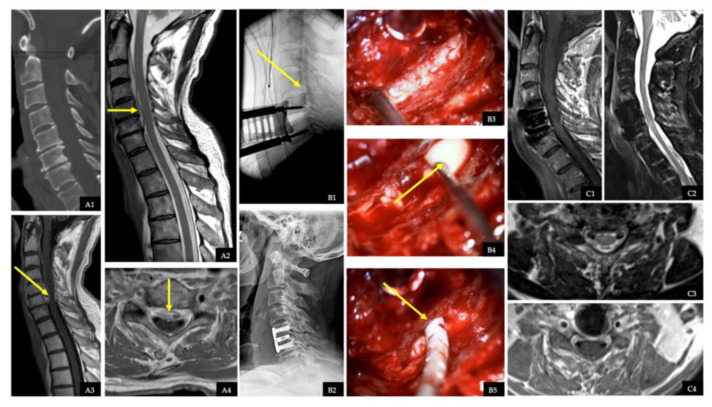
Clinical management of spinal epidural abscess. (A1): Preoperative sagittal reformatted CT image showing no sign of spondylodiscitis. (A2): Preoperative sagittal T2-weighted MR image of the cervical spine showing an isolated spinal epidural abscess (SEA) on the ventral part of the spinal canal at the level of C2 to C7 (yellow arrow). (A3,A4): Preoperative sagittal and axial contrast-enhanced T1-weighted MR images presenting the SEA (yellow arrows). (C3,C4): Preoperative sagittal and axial contrast-enhanced T1-weighted MR images of the cervical spine showing an SEA (yellow arrows). (B1): Intraoperative sagittal X-ray showing the insertion of epidural drainage from the C5/C6 level to C2 (yellow arrow). (B2): Postoperative sagittal X-ray image showing the anterior cervical discectomy and fusion (ACDF). (B3): Intraoperative image presenting the situation after discectomy of C5/C6 without signs of infection. (B4): Intraoperative image showing the pus outflow from the posterior longitudinal ligament after opening it (yellow arrow). (B5): Intraoperative image revealing the insertion of the catheter up to the level of C2 (yellow arrow). (C1–C4) Sagittal and axial T2-weighted and contrast-enhanced T1-weighted MR images at the time of discontinuation of antibiotics showing no signs of infection.
